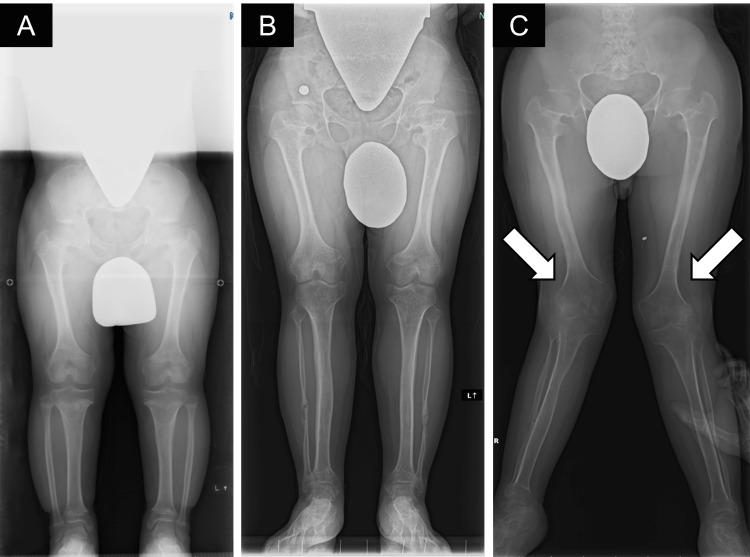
Preoperative X-rays A) Before tibia lengthening at the age of 10; B) Before femoral lengthening at the age of 13; C) After femoral lengthening. After femoral lengthening, valgus knee deformity was observed.
